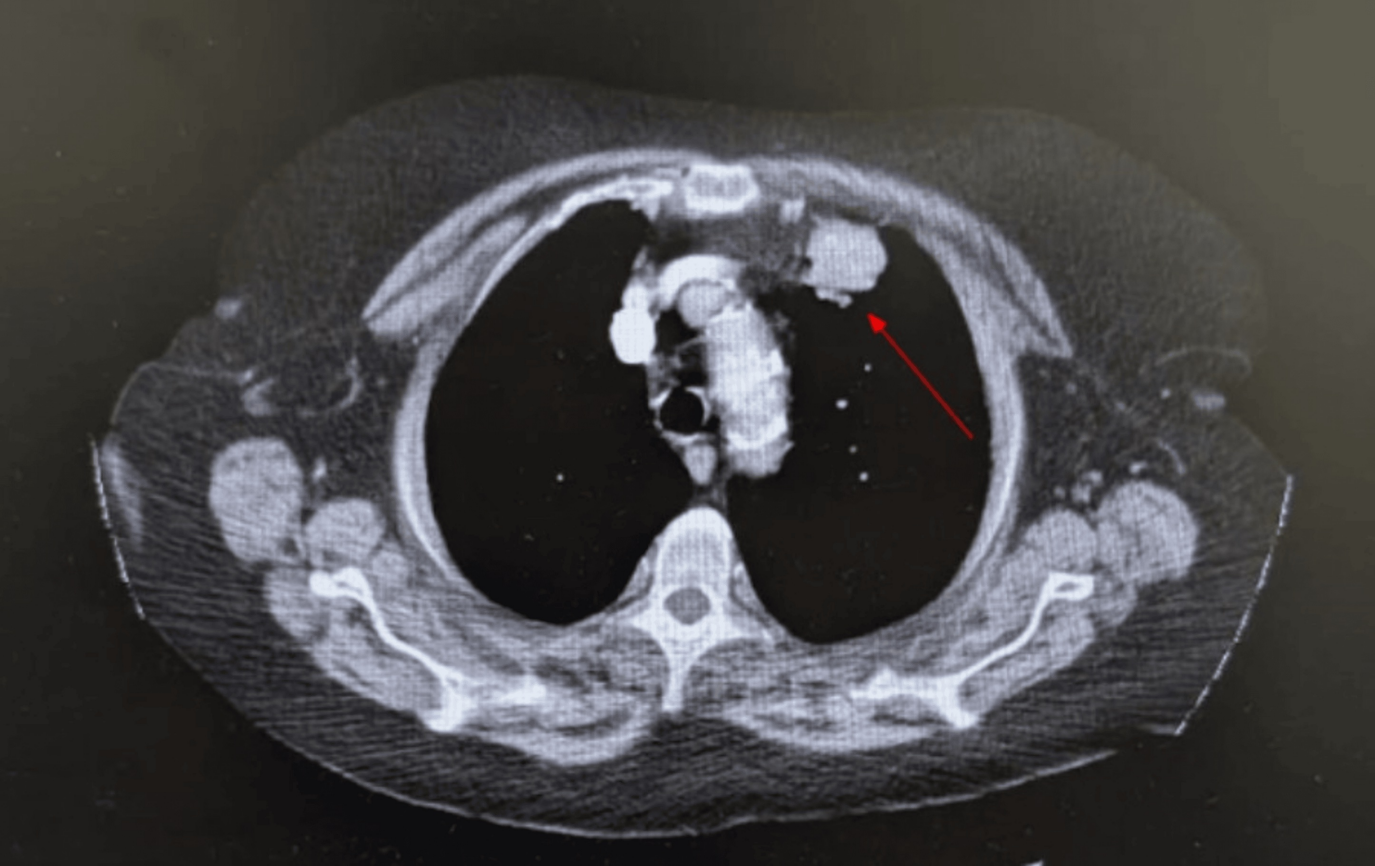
CT with IV contrast depicting lung mass Left apical lung mass measuring up to 2.9 cm. This was the presumed primary tumor based on biopsy results from the iliac lesion.
